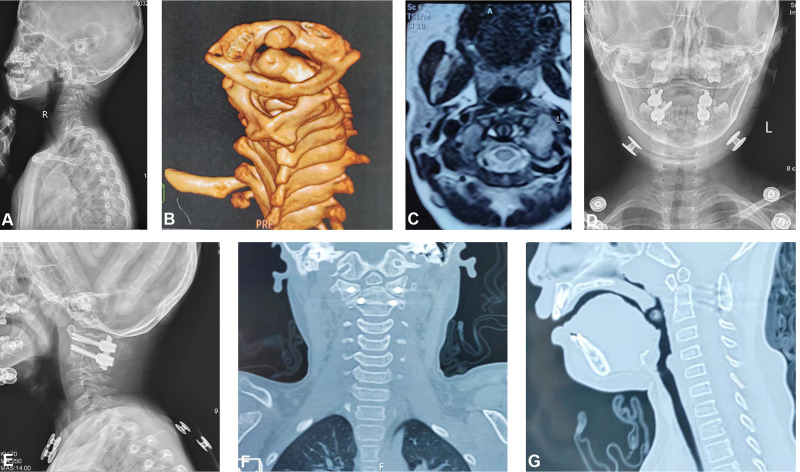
Case 4. A 4-year-old female child with DS, atlantoaxial dislocation with OsO. (A) Dynamic X-ray showed that the ADI increased significantly, and a shift of the atlas anterior and lower to the axis. (B) Three-dimensional reconstruction shows atlantoaxial dislocation with OsO. (C) MRI showed no compression of the upper cervical spinal cord. (D and E) Postoperative X-ray films showed atlantoaxial screw rod fixation. (F and G) At postoperative 1 year, atlantoaxial CT showed that the posterior atlantoaxial fusion was good, the internal fixation position was satisfactory, and there was no spinal canal stenosis.
